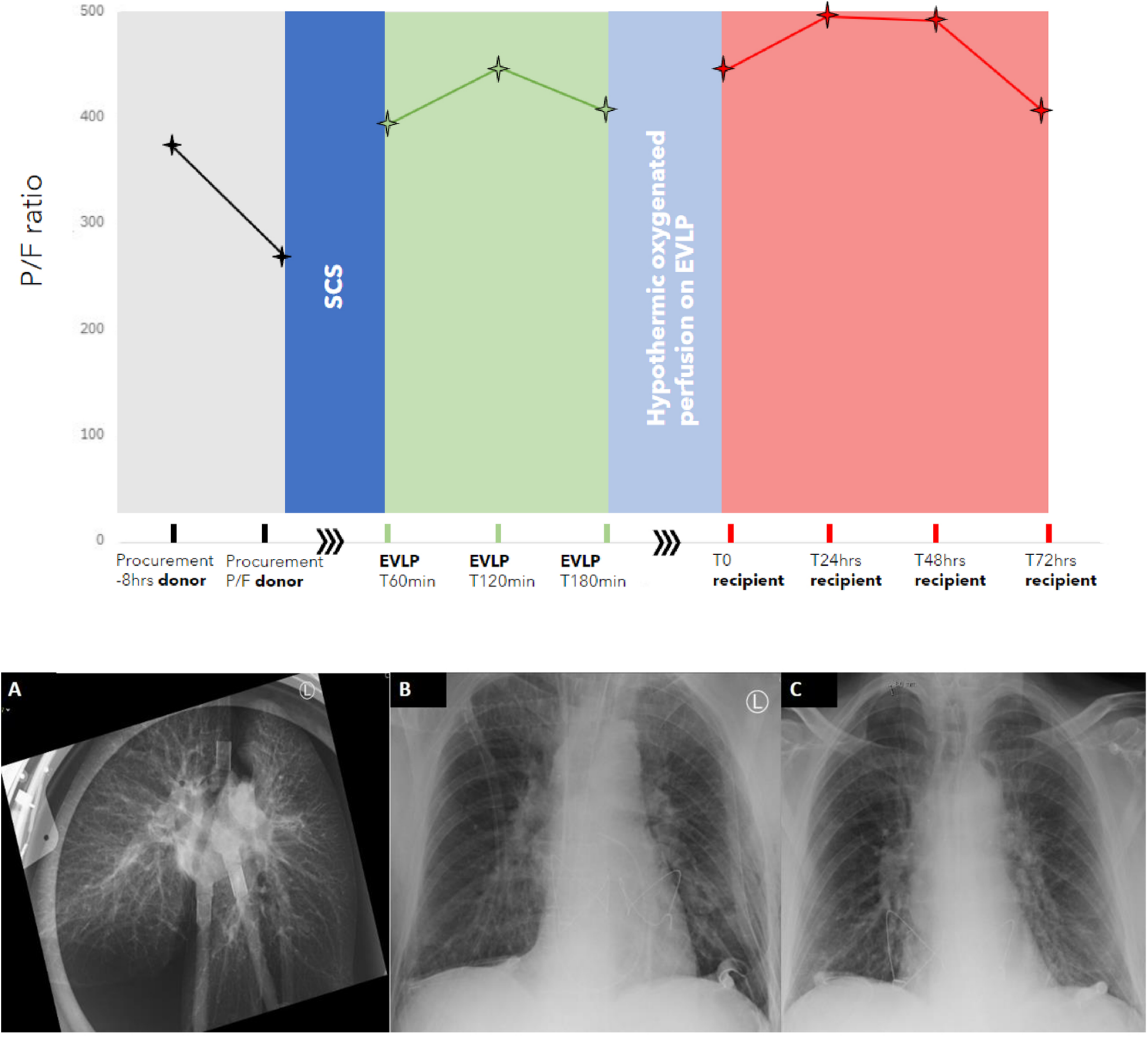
The PaO_2_/FiO_2_ ratio of the lung allografts during the transplant process from procurement in the donor to 72 h after reperfusion in the recipient. NB: The *x*-axis is not linear, the procurement and recipient phases are in hours, EVLP time is in minutes, and the post-transplant phase is in hours. SCS, static cold storage; EVLP, *ex vivo* lung perfusion. (A) X-ray at T120 mins of EVLP depicting a distribution of perfusate suggestive of hydrostatic lung edema. (B) Chest x-ray of the recipient on admission to the ICU immediately post-transplant. (C) Chest x-ray of the recipient at 72 h post-transplant.
